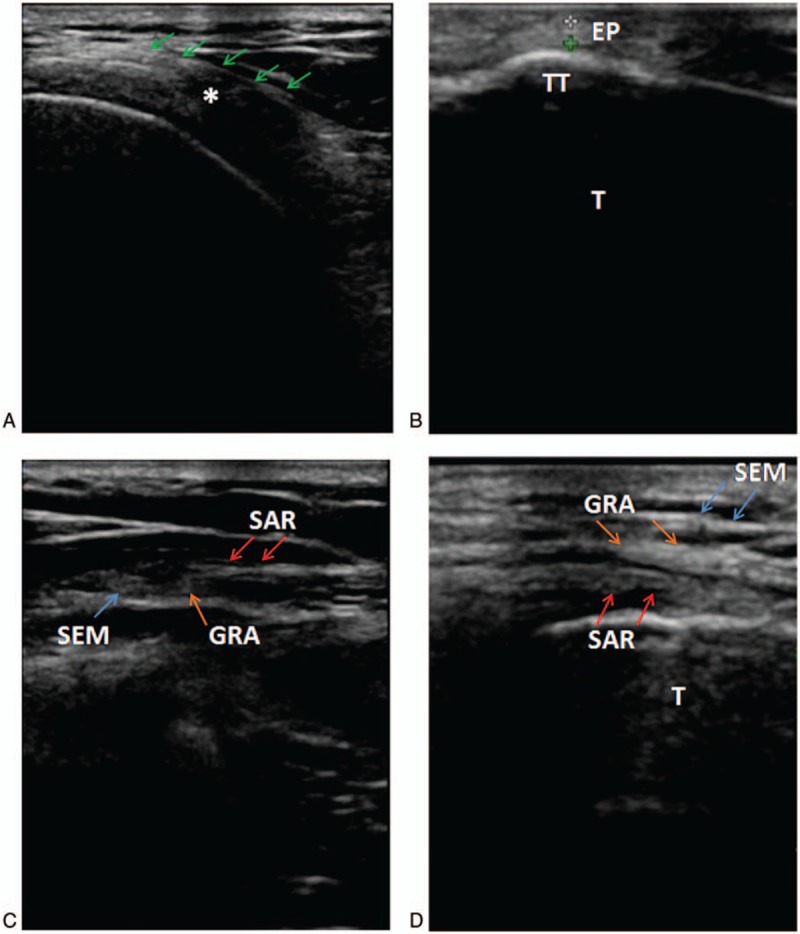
(A) ∗ = saphenous nerve, “green arrow” represented pes anserinus tendon; (B) EP = the tibial attachment of pes anserinus (among the white asterisk and green asterisk), T = tibia, TT = tibial tuberosity; (C) ultrasonic cross-section image. Type II arrangement of pes anserinus tendon: sartorius tendon covered gracilis tendon and incompletely covered semitendinosus tendon. GRA = gracilis tendon, SAR = sartorius tendon, SEM = semitendinosus tendon; (D) ultrasonic longitudinal section image. Type I arrangement of pes anserinus tendon: the tendon of sartorius did not cover the tendon of gracilis. (This image was scanned from the superior.) GRA = gracilis tendon, SAR = sartorius tendon, SEM = semitendinosus tendon, T = tibia.
